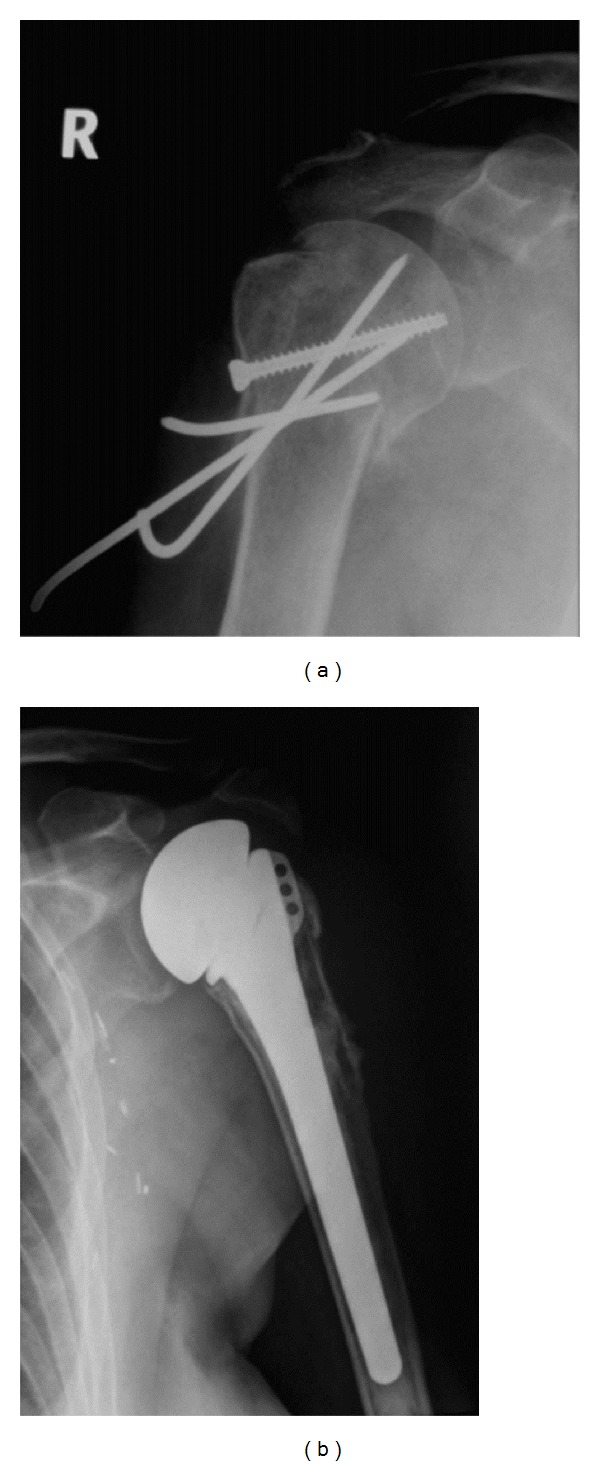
Postoperative radiographs demonstrating internal fixation with 2.7 mm threaded wires and figure of 8 tension band sutures using number 5 Ethibond suture on the right side and a hemiarthroplasty on the left side.
